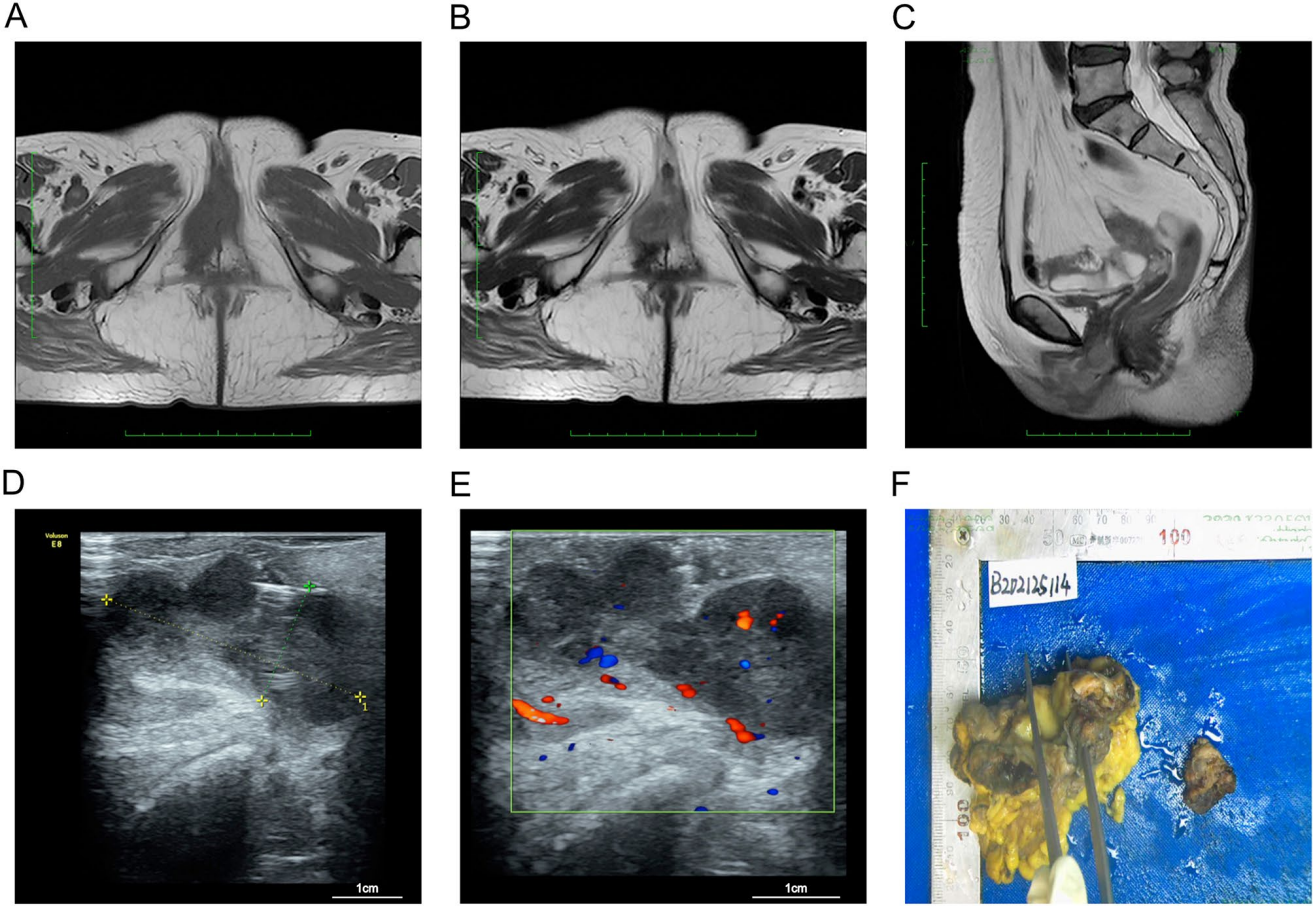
Clinical images of the patient. (A) T1 MRI transverse scanning images of vulvar melanoma in the patient; (B) T2 MRI transverse scanning images of vulvar melanoma in the patient; (C) T1 MRI longitudinal scanning images of vulvar melanoma in the patient; (D) Ultrasound image of vulvar melanoma in the patient; (E) Doppler images of enriched blood supplements in vulvar melanoma; (F) Sample of vulvar melanoma after surgical removal
